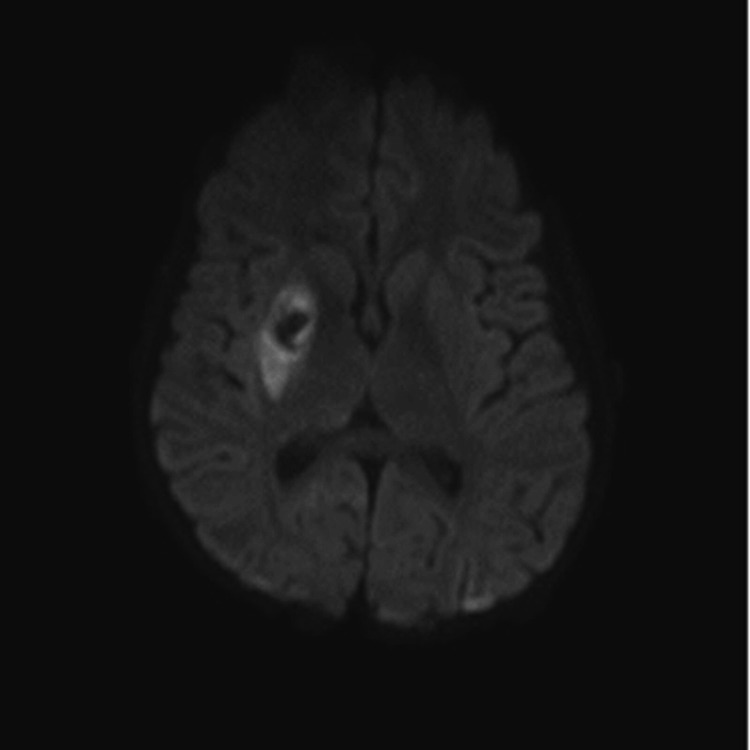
MRI image of ischemic infarct of right basal ganglia with hemorrhagic transformation
